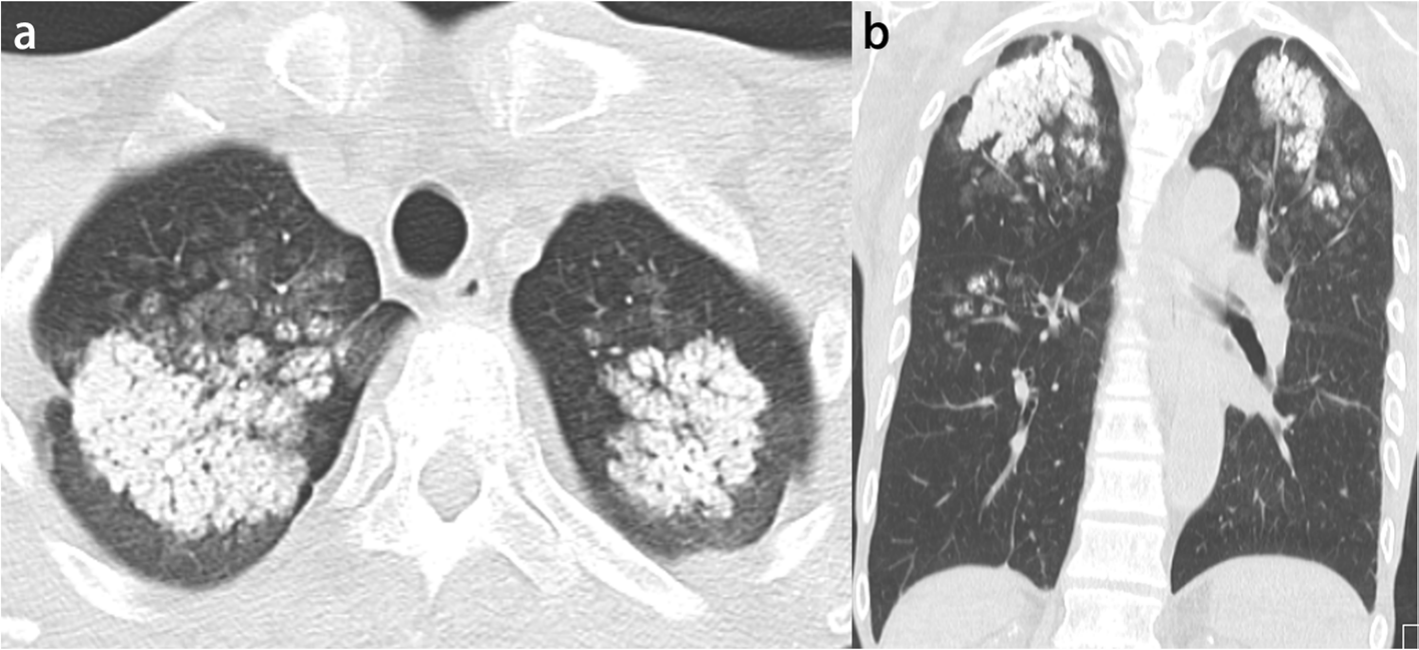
Metastatic calcification in a 61-year-old man. a CT image shows mass-like opacities containing dense calcification in the bilateral upper lobes. b Coronal CT image reveals that calcification is predominantly distributed in the upper lobes
